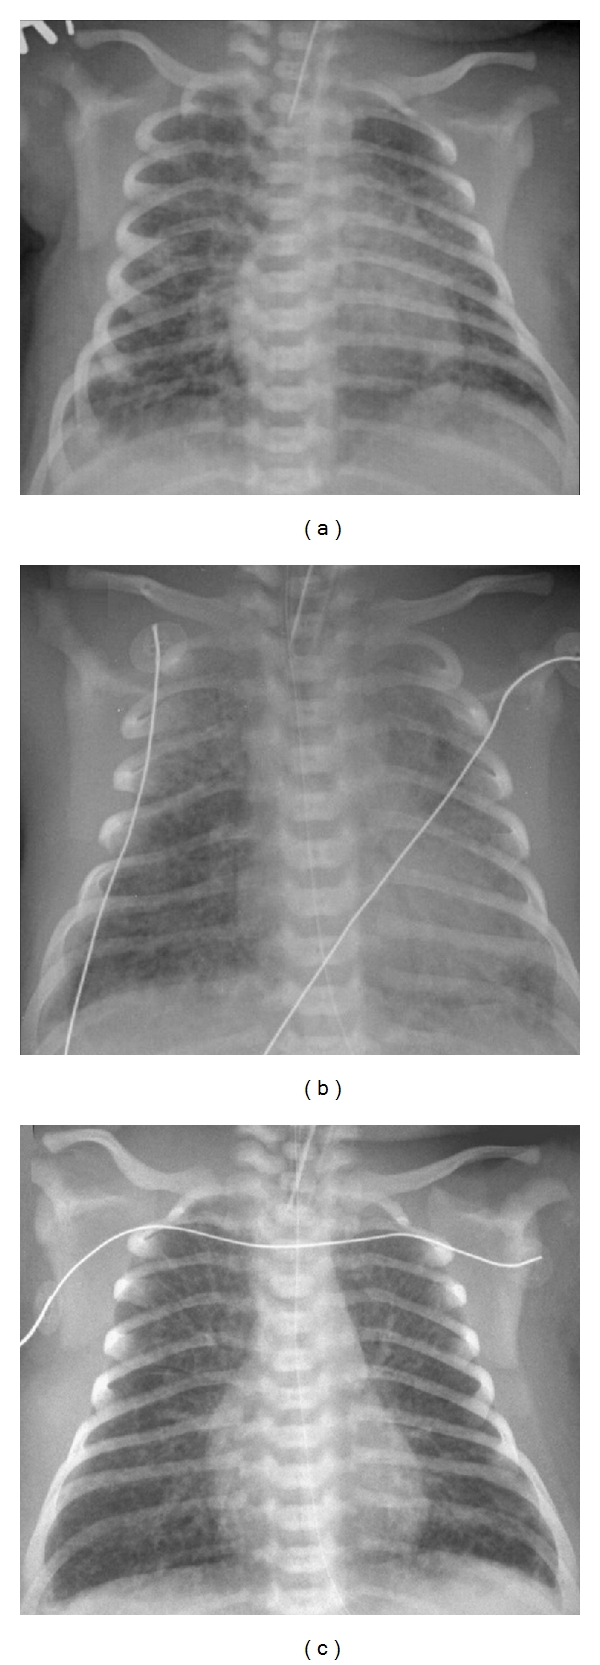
Chest X-ray appearances in ventilated infants with MAS. (a) Typical appearance of MAS showing “fluffy” opacification widespread throughout the lung fields. (b) Marked atelectasis in an infant with profound hypoxaemia. (c) Hyperinflation and gas trapping, with a narrow cardiac waist, flattened diaphragms, and intercostal bulging of the lung.
